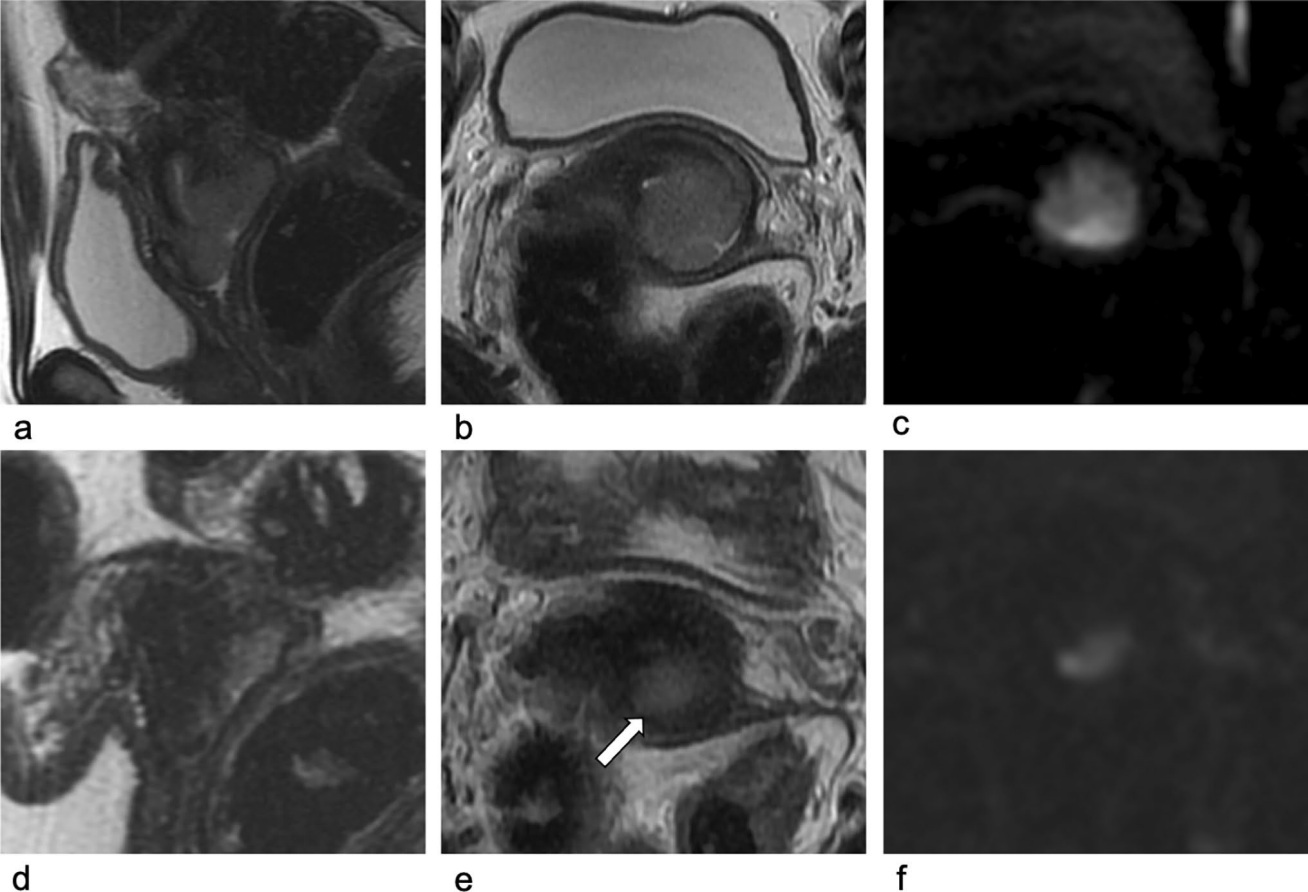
Baseline and post-NACT MRI in 26-year-old woman with cervical adenocarcinoma (FIGO IB2). a, d Sagittal T2-w images. b, e Axial oblique T2-weighted images. c, f Axial oblique DWI images (b value 800 s/ mm^2^). At baseline MRI (a, b, c), T2-w sequences show the hyperintense cervical mass, extending to the upper third of the vagina, with maximum diameter of 30 mm (any plane). The lesion shows high signal intensity also on DWI sequence (c). After NACT (d, e, f), T2-w sequences show the presence of an area of hyperintensity in the cervix (arrow in e), to which corresponds to a high-signal-intensity area also on DWI images (f), configuring a PR. NACT: neoadjuvant chemotherapy; PR: partial response
